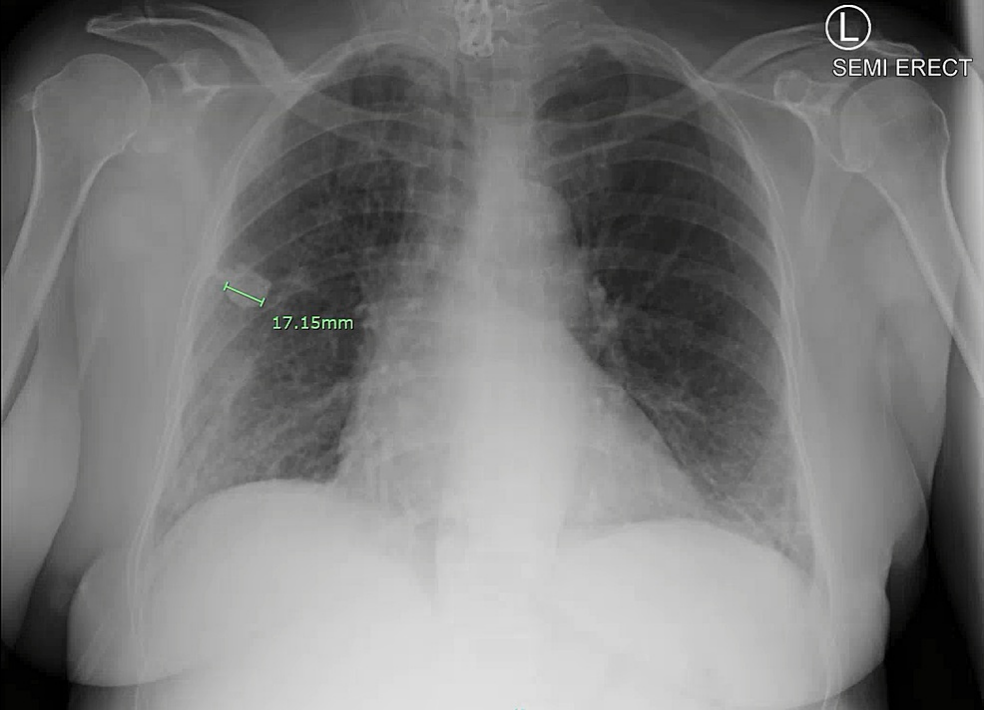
Repeat Chest X-ray 2 Days After Treatment
